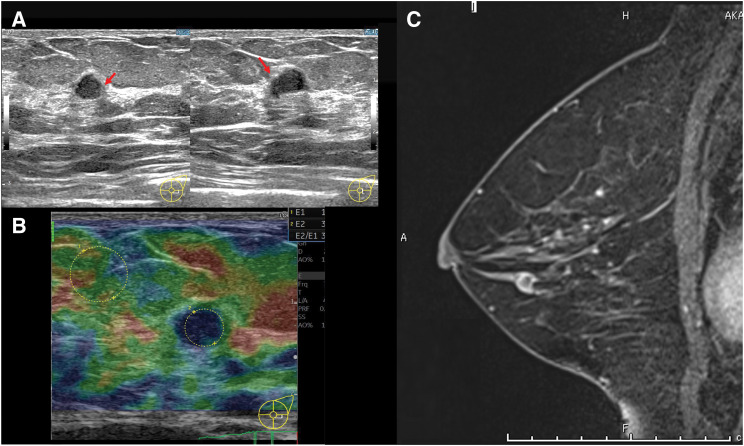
Imaging findings of Case 2. (A) B-mode US demonstrates a 7-mm cystic mass with a papillary internal component (arrows) and (B) a Tsukuba elasticity score of 4. (C) MRI shows a small cystic tumor with a markedly enhanced thickened wall and heterogeneous internal enhancement. US, ultrasonography
